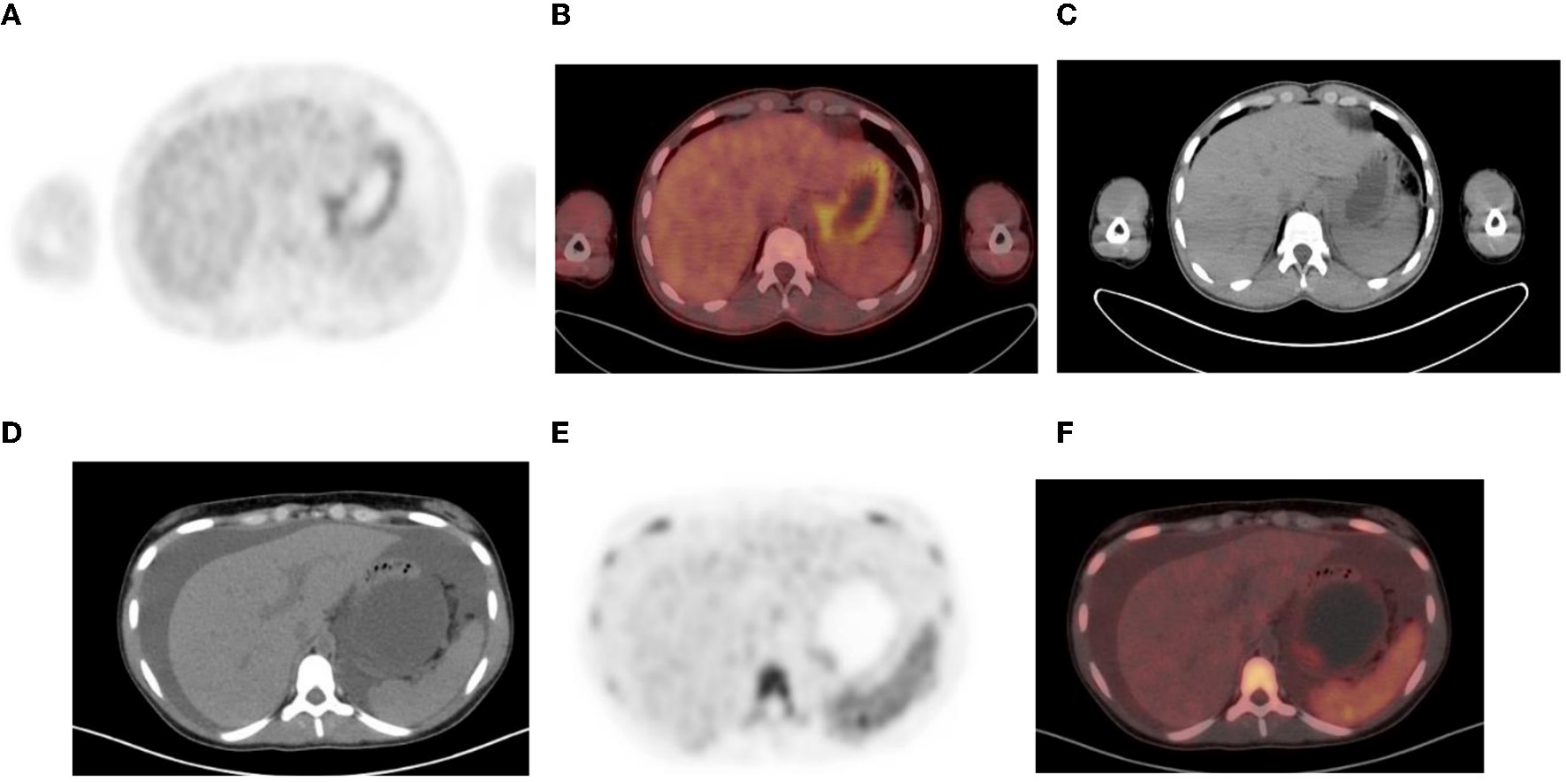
(A–C) Normal physiological liver to spleen ratio. Physiological liver uptake is always more than the spleen uptake, except in certain pathologies like lymphomatous involvement of the spleen, HIV or in patients who are post chemotherapy or after the administration of granulocyte colony stimulating factor. Note the physiological the uptake in the gastric wall in a contracted stomach. Note that in (A) and (C), non-contrast CT has a limited sensitivity in showing splenic lesions, however the uptake in the spleen is greater than the physiological liver uptake and the presence of splenomegaly is compatible with pathologic involvement. (D–F) Diffuse increased uptake in the spleen, greater than the physiological liver uptake in a 10-year-old female with diffuse large B cell lymphoma (DLBCL) prior to chemotherapy. Staging PET/CT showed stage 4 disease with nodal disease above and below the diaphragm, bone marrow and splenic involvement. (B) PET and (C) fused PET/CT showing increased uptake in the spleen and bone marrow compared to the physiological uptake in the liver. Note that in (F), a non-contrast CT has a limited sensitivity in showing splenic lesions, however the uptake in the spleen is greater than the physiological liver uptake and the presence of splenomegaly is compatible with pathologic involvement.
